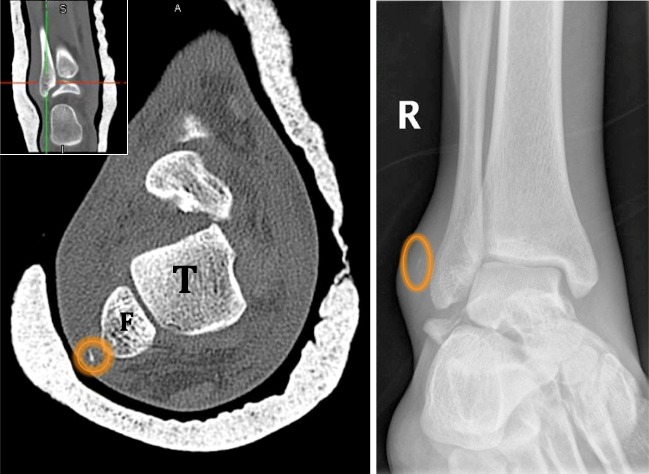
Axial CT cut (Fig. 2a) and internal rotation ankle plain X-ray (Fig. 2b) showing talar neck fracture and fleck sign (circled)
